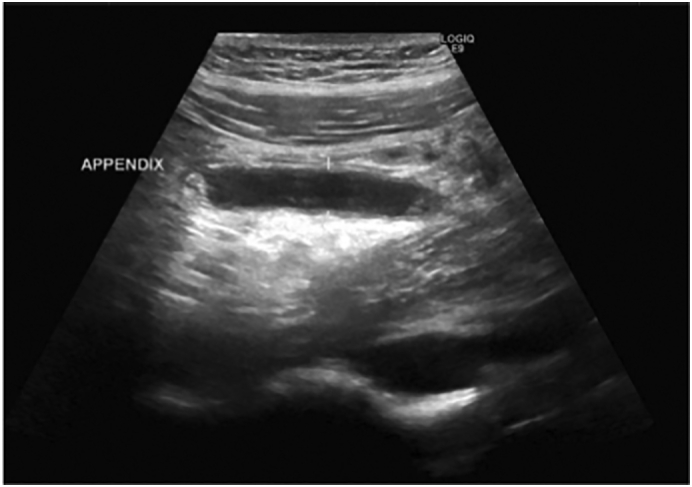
Ultrasonic image showing non-compressible structure at the RIF (Appendix).
